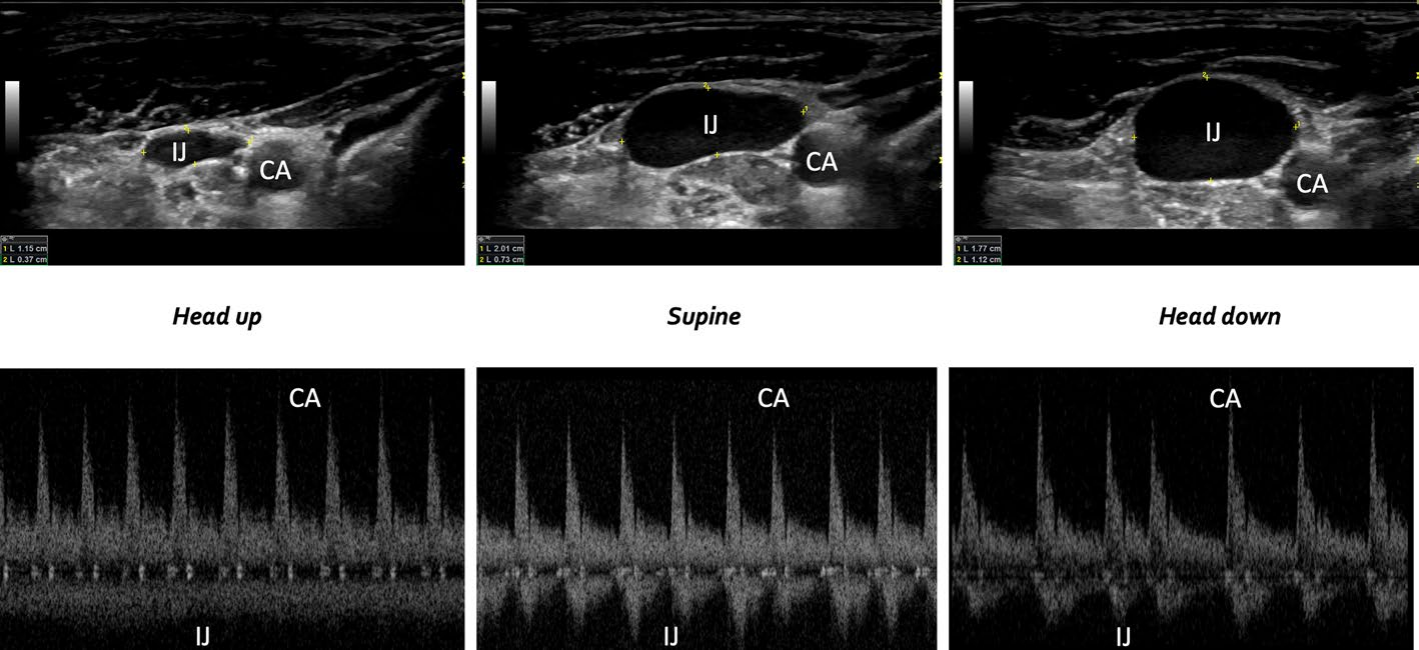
The relationship between B-mode images and Doppler spectra at three preload conditions in a single subject. CA is carotid artery and IJ is internal jugular
